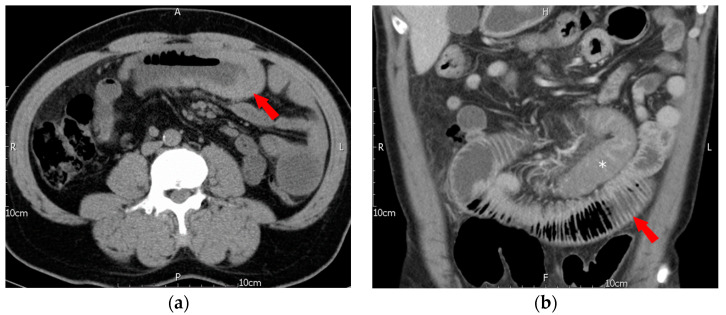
Second episode of intramural hematoma of jejunum. (a) Axial non-contrast CT showed segmental thickening of the midjejunum with diffuse hyperdensity, indicating intramural hematoma (arrow), (b) coronal reformatted contrast-enhanced CT showed intramural hematoma (*) of the midjejunum and marked dilatation of the proximal jejunum, indicating mechanical ileus (arrow).
